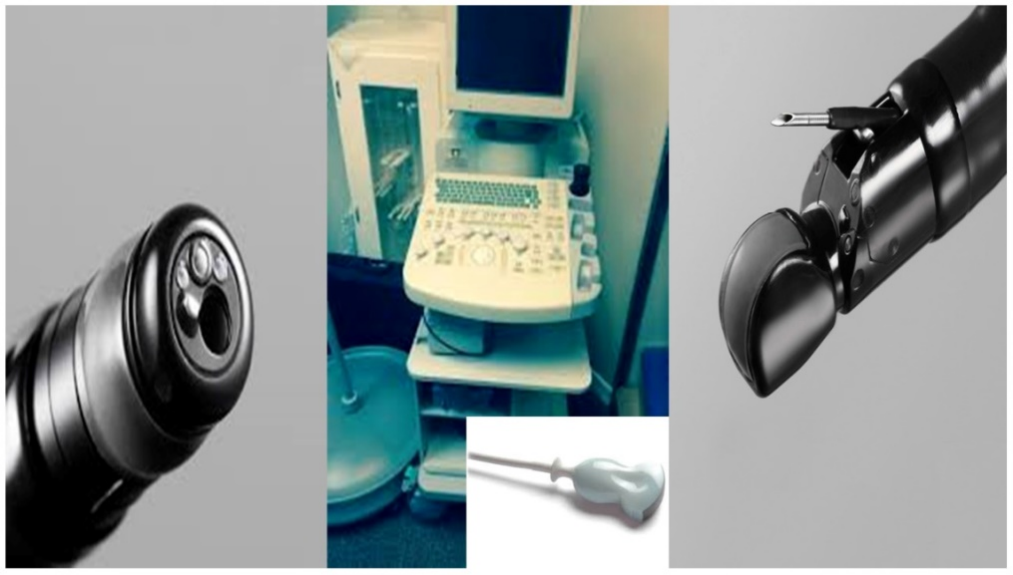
From left to right; Radial esophageal ultrasound, convex probe and convex probe esophageal ultrasound
